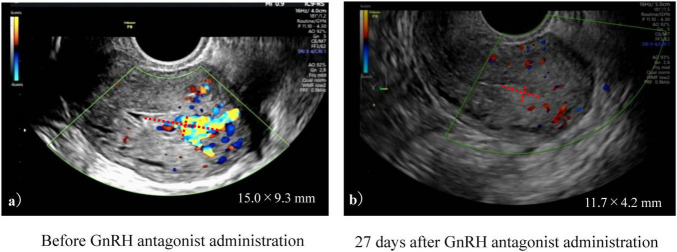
A representative imaging of retained products of conception (RPOC) in transvaginal ultrasound (Case 10, Table 1). (a) RPOC imaging before taking an oral Gonadotropin-Releasing Hormone (GnRH) antagonist. (b) RPOC imaging after taking an oral GnRH antagonist for 27 days. Red dotted lines show the long and short diameters of RPOC.
